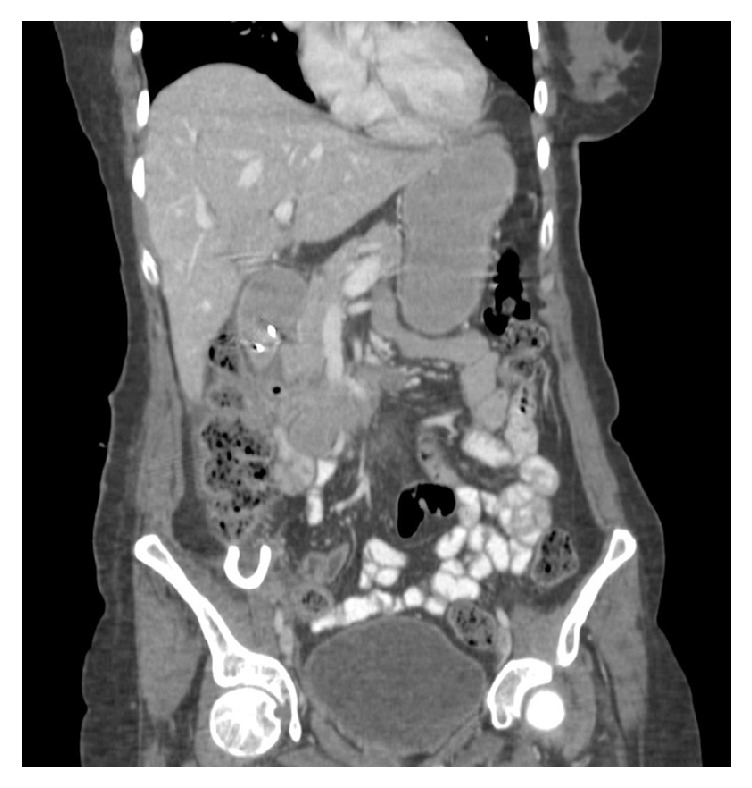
Coronal abdominal CT scan images after the closure of the fistula.
